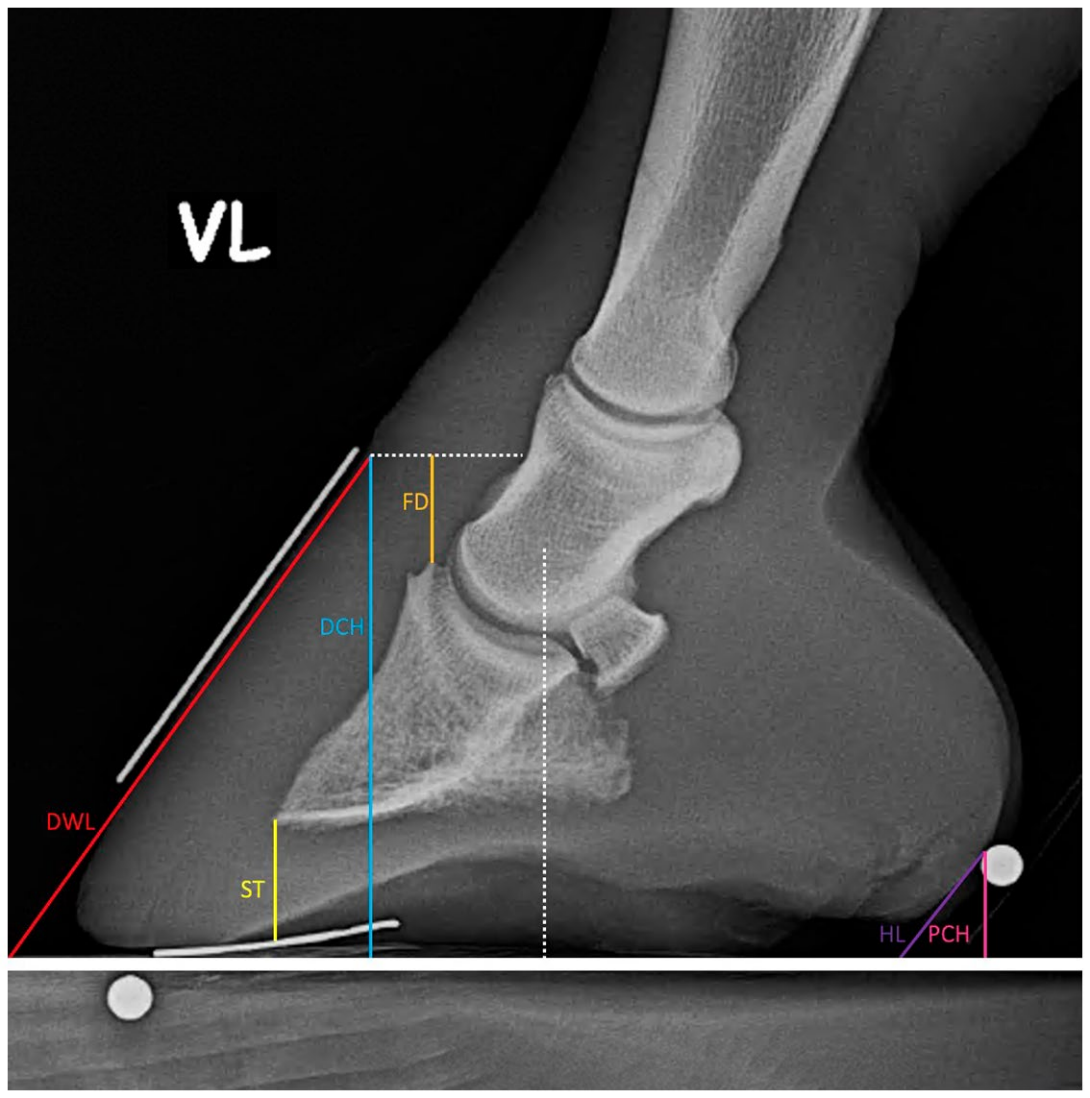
Length measurements on the lateromedial X-Ray.
